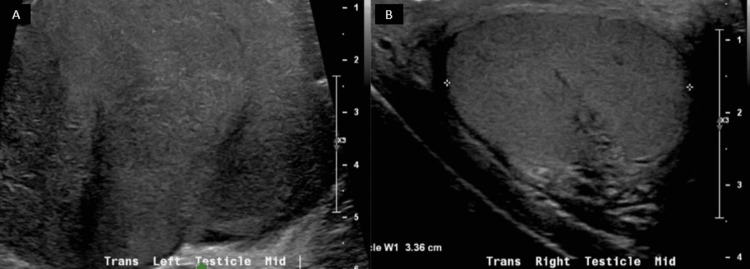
Scrotal ultrasound showing a lobulated, irregular left testicle (A) and normal right testicle (B)
